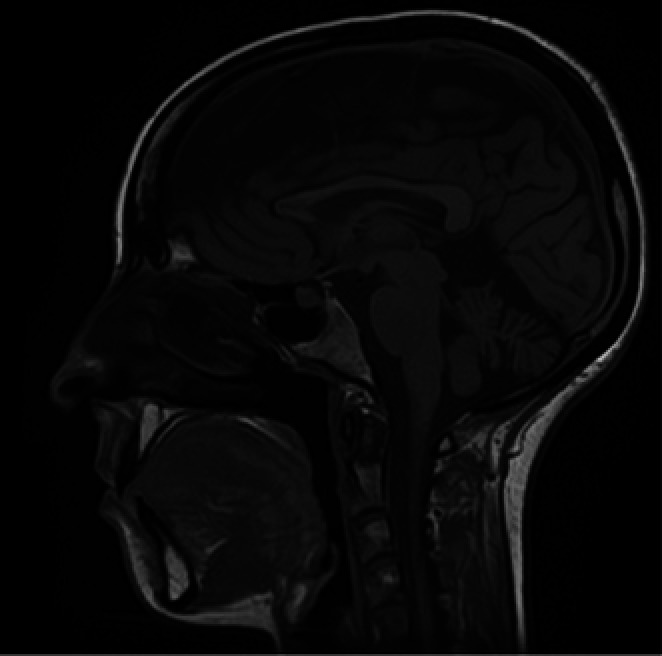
FIGURE 2 Brain MRI (sagittal T1) obtained at age 34 demonstrating thinning of the corpus callosum, bilateral enlargement of frontal pericortical spaces, and mild widening of pericerebellar cisterns.
